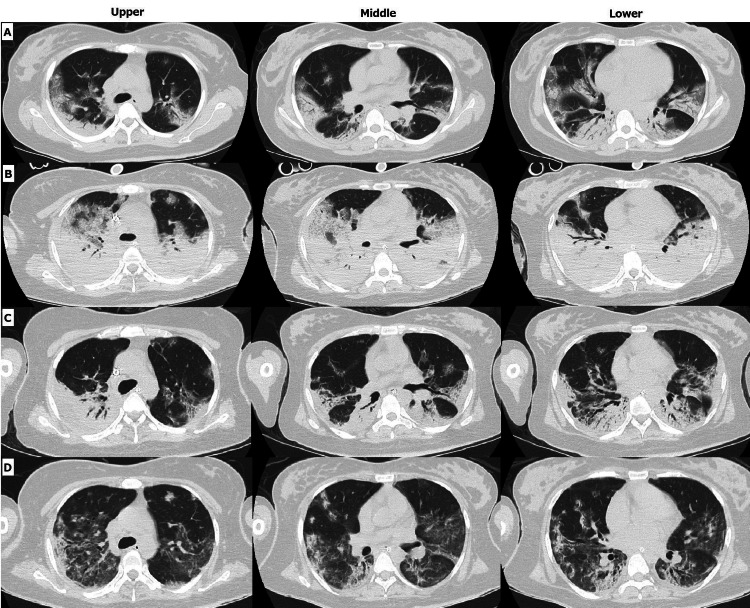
Chest computed tomography (CT) of the patient A. Chest CT on admission (28 weeks, 0 days). Pulmonary consolidation was observed in bilateral dorsal lungs. B. Chest CT on initiation of VV-extracorporeal membrane oxygenation (ECMO) (POD1). Diffuse-distributed ground-glass shadows in the bilateral lung fields were observed. The P/F ratio worsened, requiring VV-ECMO. C. Chest CT on laparotomy (POD9). The ground-grass shadows in the bilateral lungs tended to be reduced. D. Chest CT 1 day before extubation (POD15). The ground-glass shadows in the bilateral lungs were mostly absorbed. CT, computed tomography; VV, venovenous; POD, postoperative day
